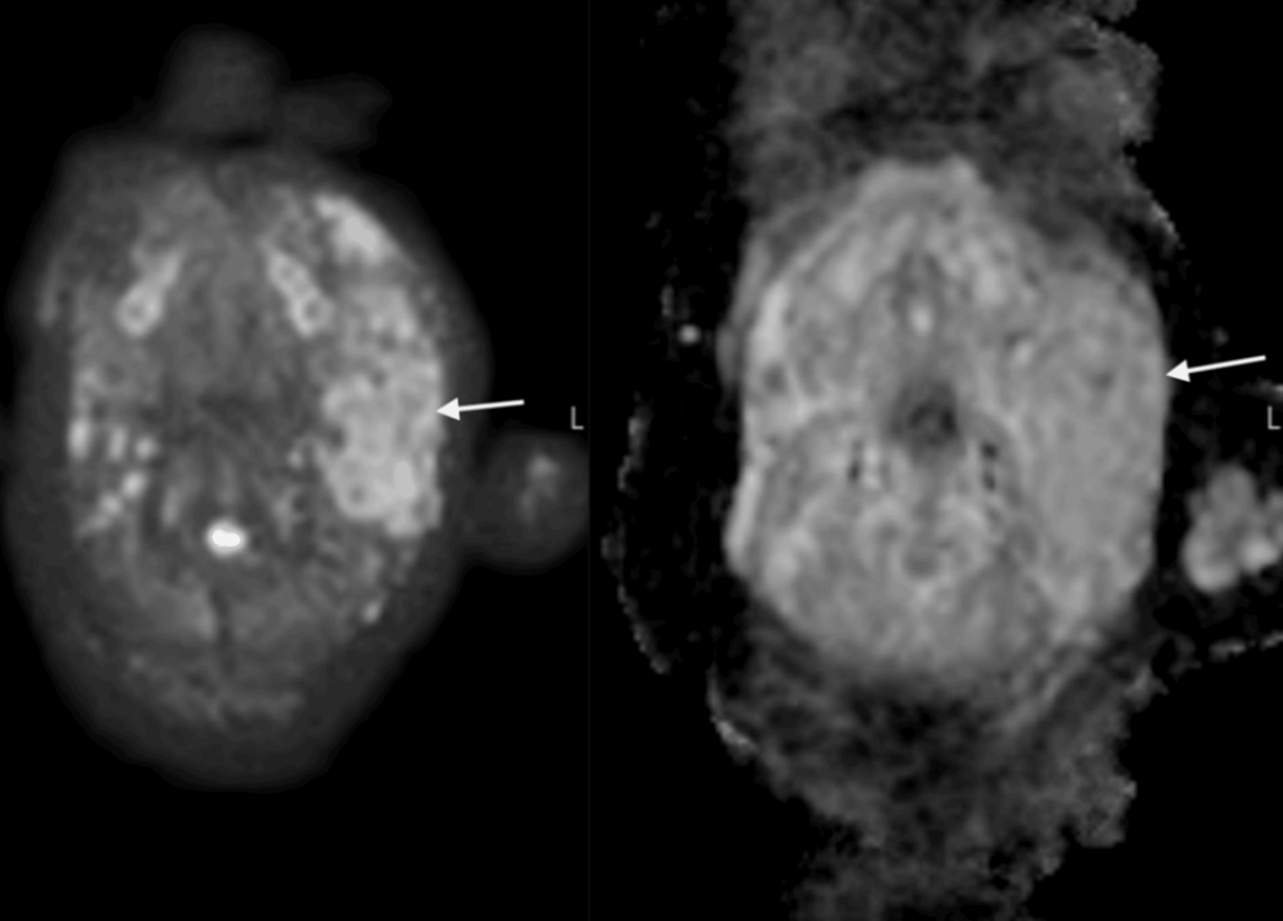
DWI shows T2 shine-through phenomenon of the left enlarged parotid (white arrows).
